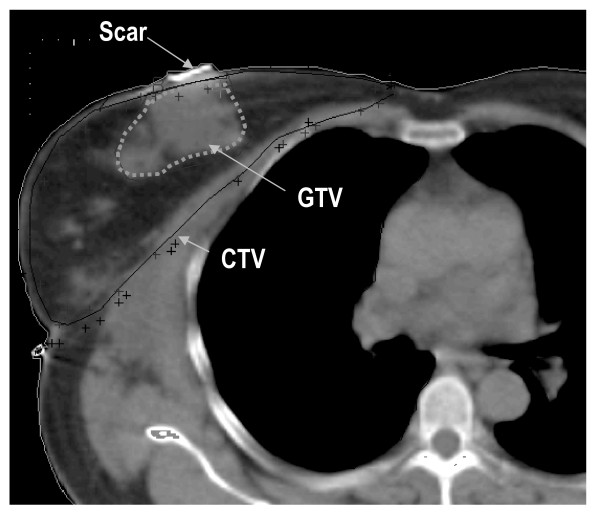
Target volume definition on CT.
